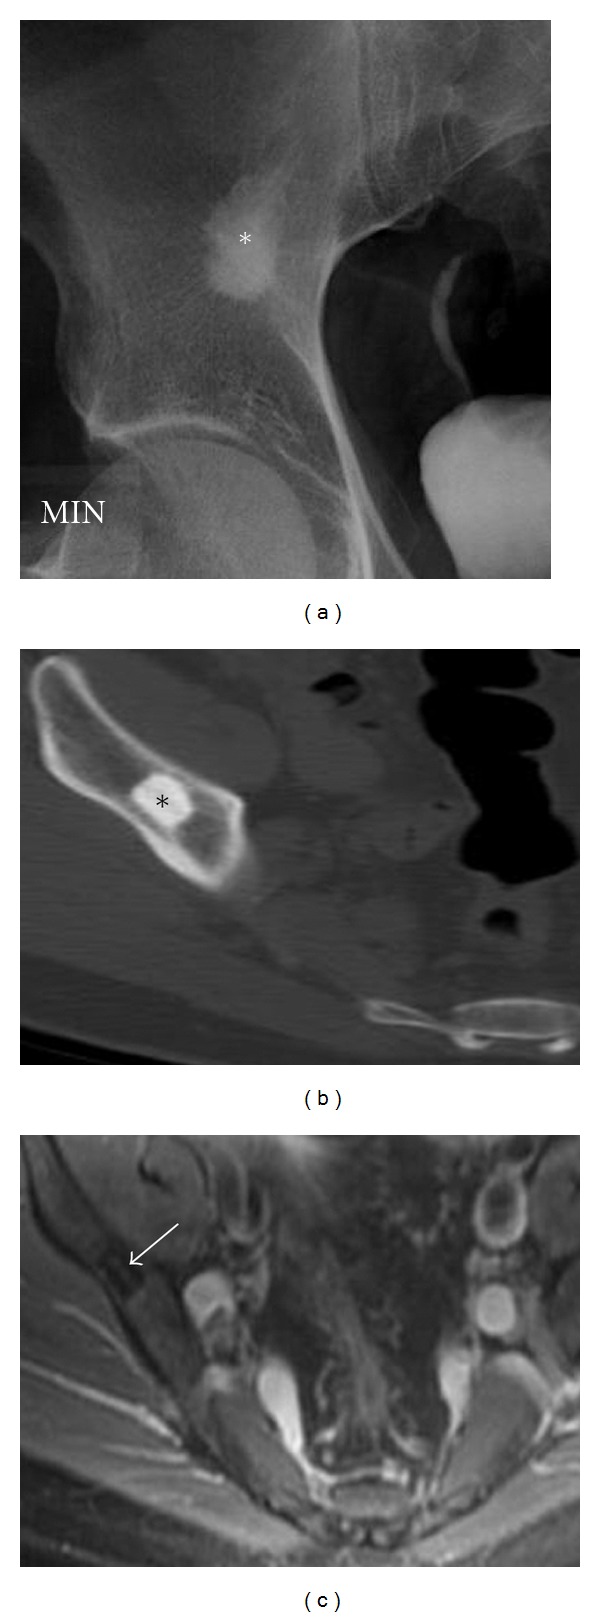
Bone island. Incidental right ilium lesion noted in a 47-year-old patient presenting with hematuria diagnosed as a bone island. AP radiograph (a) depicts a large sclerotic lesion with speculated margins blending with surrounding trabecula, a characteristic finding of a bone island (enostosis). A bone island is cortical bone in the medullary cavity (b) and hence is of low signal in all MR sequences including axial T2 MRI (c).
